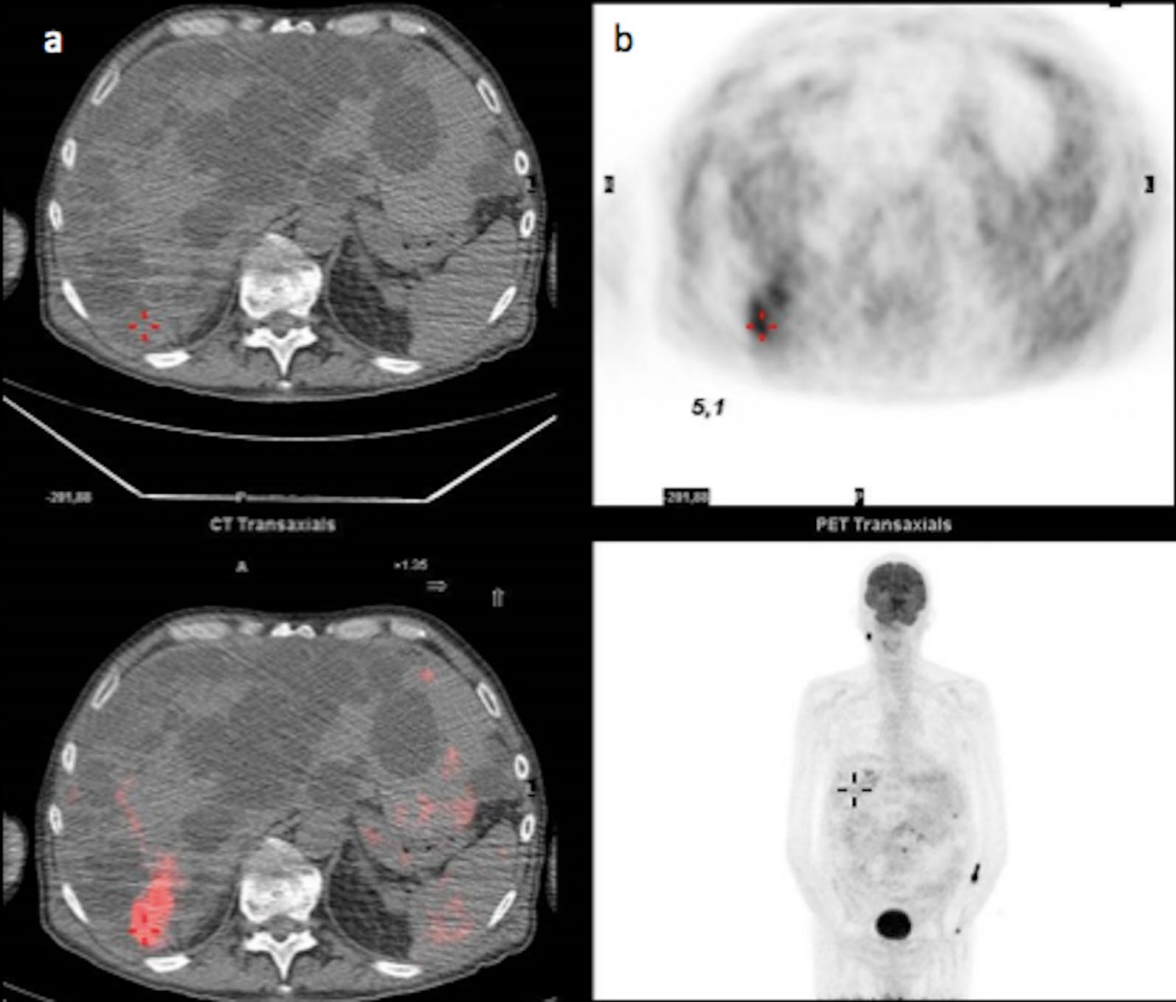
Infected cyst in a 65-year-old male patient with autosomal dominant polycystic liver disease. a Axial non-enhanced computed tomography depicts one more attenuating cyst among multiple simple cysts (cross); b 18-F-FDG PET imaging shows the increased metabolism of that cyst (SUV at 5,1)
